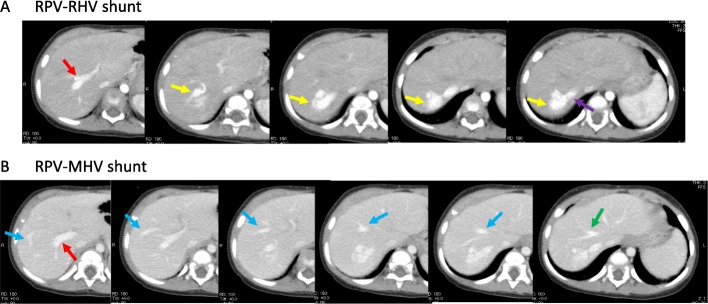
Enhanced computed tomography at 1 year of age. a Enhanced computed tomography (CT) revealing a shunt vessel (yellow arrow) between the right portal vein (RPV) (red arrow) and right hepatic vein (RHV) (purple arrow). The shunt vessel (yellow arrow) presented a nidus-like form. b Enhanced CT revealing a shunt vessel (blue arrow) between the right portal vein (RPV) (red arrow) and the middle hepatic vein (MHV) (green arrow)
